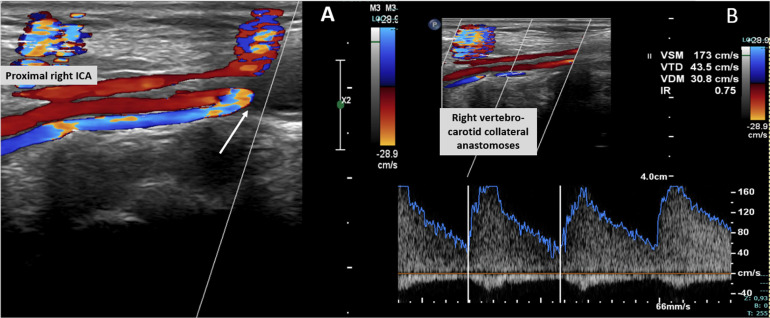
Duplex ultrasound of the right cervical carotid axis demonstrating proximal occlusion of the internal carotid artery with retrograde collateral inflow reconstituting the cervical ICA segment (white arrow) (A). A vertebro-carotid collateral vessel is identified; its direction and morphology are compatible with a persistent embryologic carotid–vertebrobasilar anastomosis, most likely a persistent hypoglossal artery or proatlantal intersegmental artery (white arrow). Spectral Doppler (B) shows high-velocity, low-resistance flow (PSV 173 cm/s, EDV 43.5 cm/s; RI 0.75), consistent with a hemodynamically significant collateral supplying the distal ICA territory.
